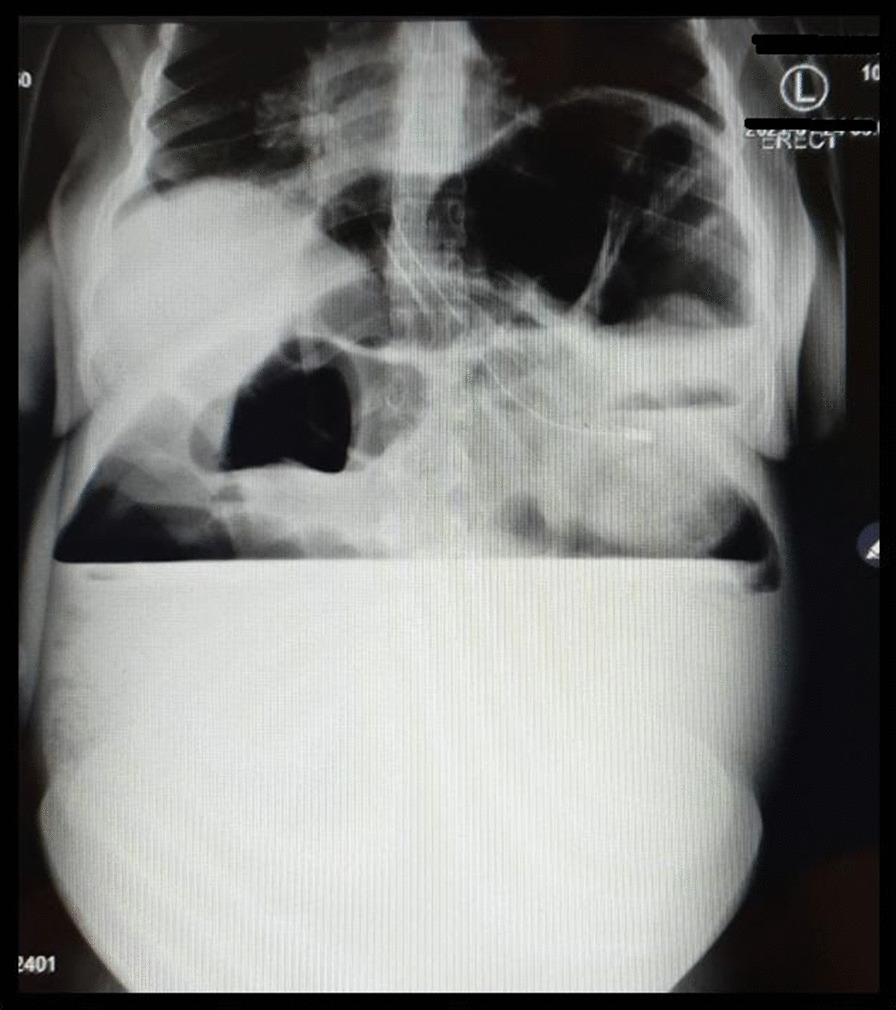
Erect abdominal X-ray showing high air–fluid level and distended gastric bubble
